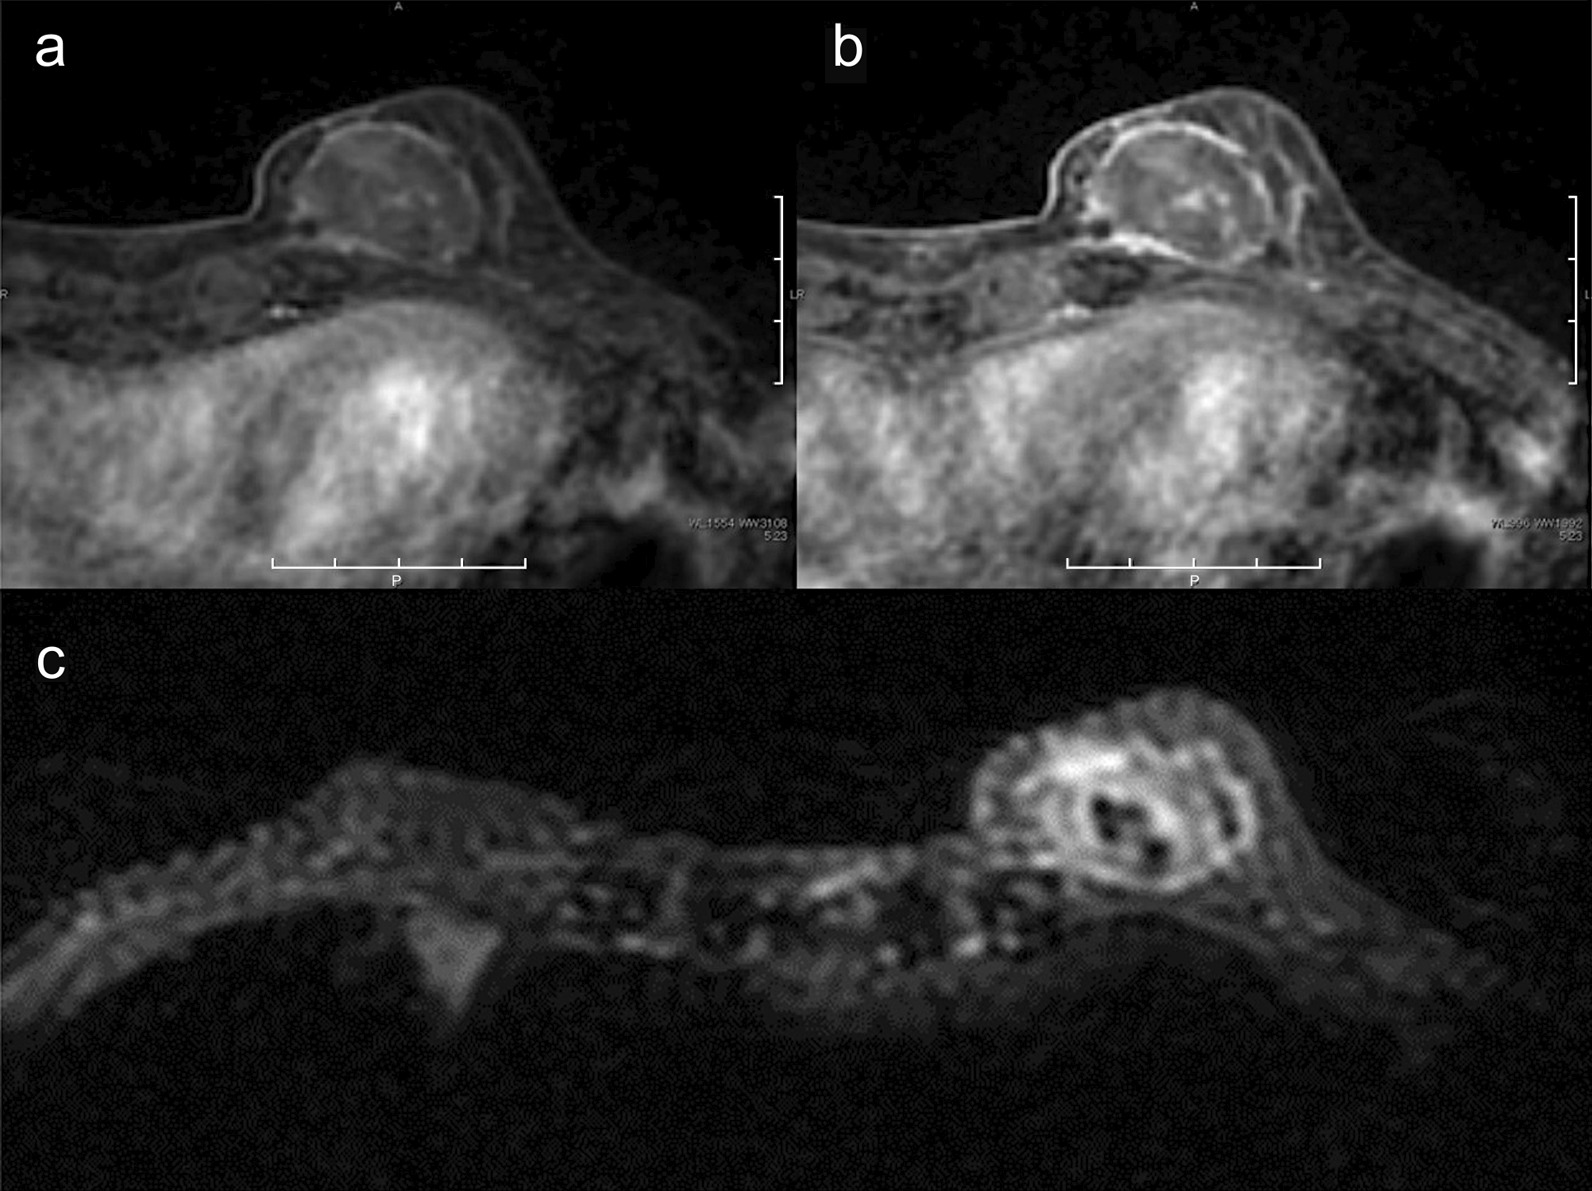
Contrast-enhanced breast magnetic resonance imaging (MRI). a Initial phase of dynamic contrast-enhanced MRI, b Delayed phase of dynamic contrast-enhanced MRI; the contrast effect appeared only on margins and part of the interior, c Fat-suppressed T2 weighted image; the mass showed high signal
